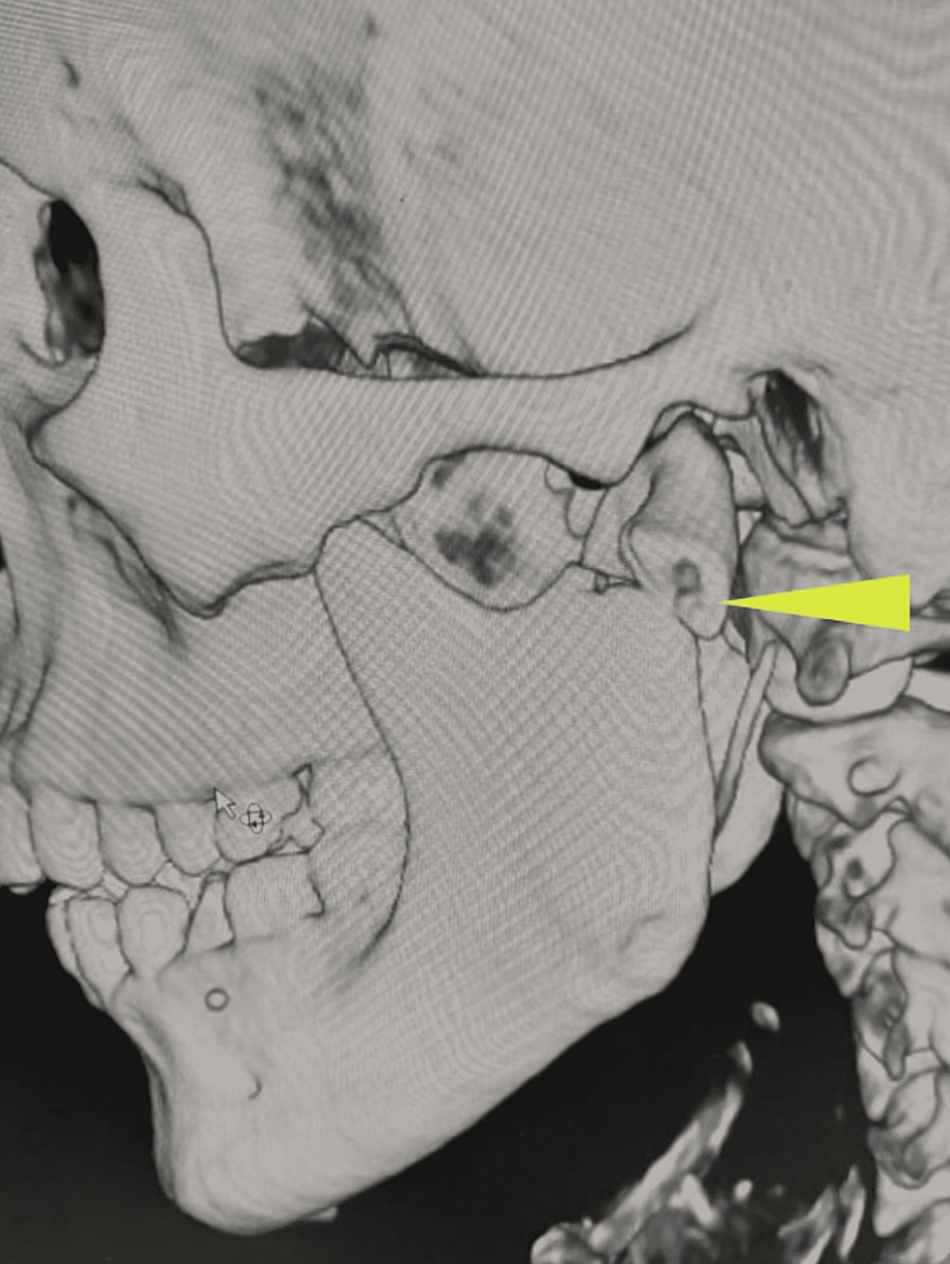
Three-dimensional computed tomography scan revealing a medially dislocated left mandibular condylar fragment (arrow)
